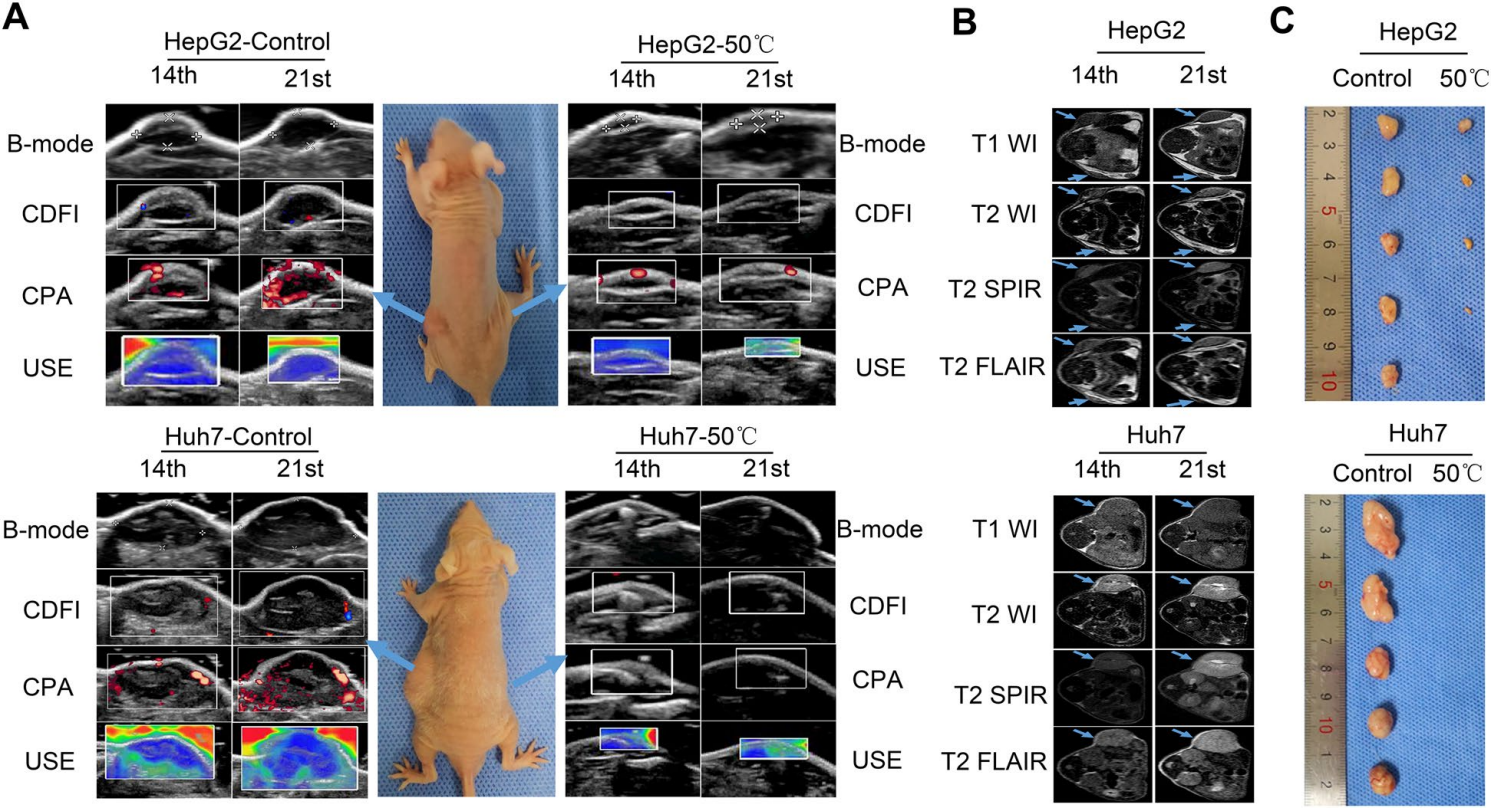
Hyperthermia inhibits growth of HCC in vivo. A Representative images of tumors implanted with HepG2 and Huh7 cells by US imaging at the 14th and 21st day. (Left panel represents the control and right panel represents the 50 °C treatment, and arrows indicate US imaging of the corresponding tumors). B Representative images of tumors by MRI at the 14th day and 21st day. The arrows indicating the location of tumors. C The photographs of excised tumors at the 21st day
